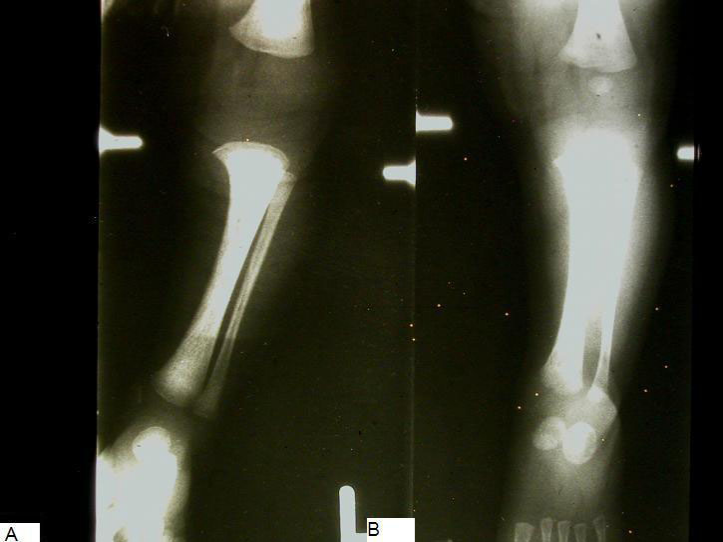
Radiograph of the left lower extremity of two infants, showing absence of the distal femoral epiphysis on left. Radiograph of the left lower extremity of two infants. The infant on the left with congenital hypothyroidism demonstrates absence of the distal femoral and proximal tibial epiphyses, while in the normal infant on the right the distal femoral epiphysis is present.
